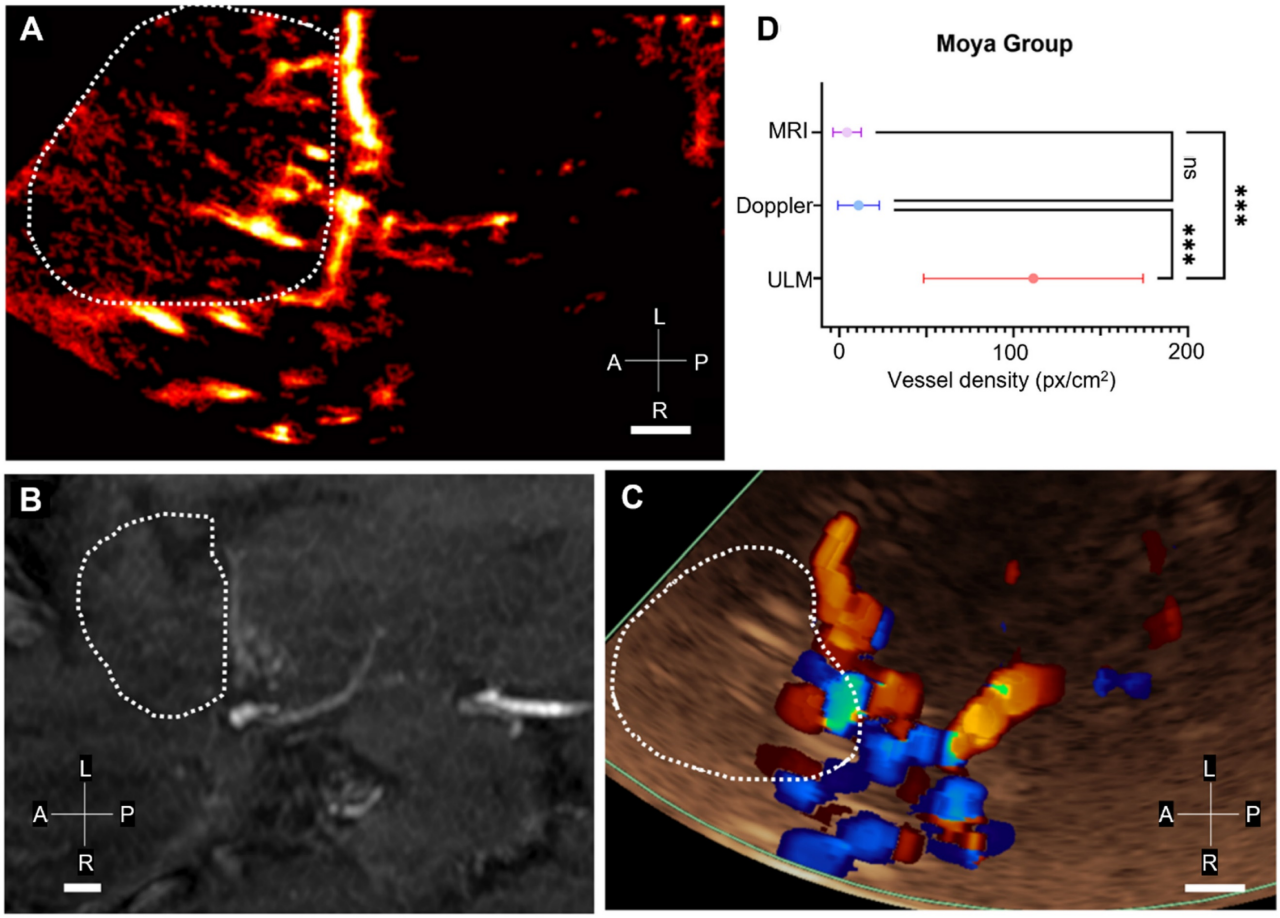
Comparison of vessel density in MRI, ULM, and color Doppler in Moyamoya patients. A) ULM density map on the patient n°16 (Moyamoya group). White dotted lines indicate perforating arteries' area segmentation, B) MRI TOF 3T of the same patient (axial view). White dotted lines indicate perforating arteries' area segmentation, C) Color doppler of the same patient. White dotted lines indicate perforating arteries' area segmentation, D) Comparison of vessel density, i.e. number of pixels superior to the median intensity of the image, between the three techniques. One-way ANOVA test (see Methods). Scale bars of 1 cm. Radiological notation, P: Posterior, A: Anterior, R: Right, L: Left.
